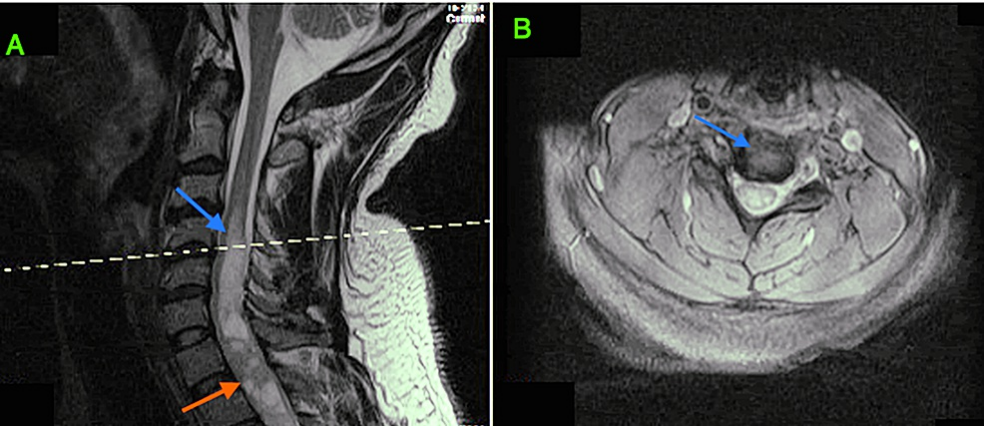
T1-weighted MRI cervical spine. (A) Large syrinx at the C4-C7 (blue arrow). A heterogeneous expanded cord with cystic changes at the C7-T1 (red arrow). (B) Axial cross-sectional cervical spine at C4-C5.
